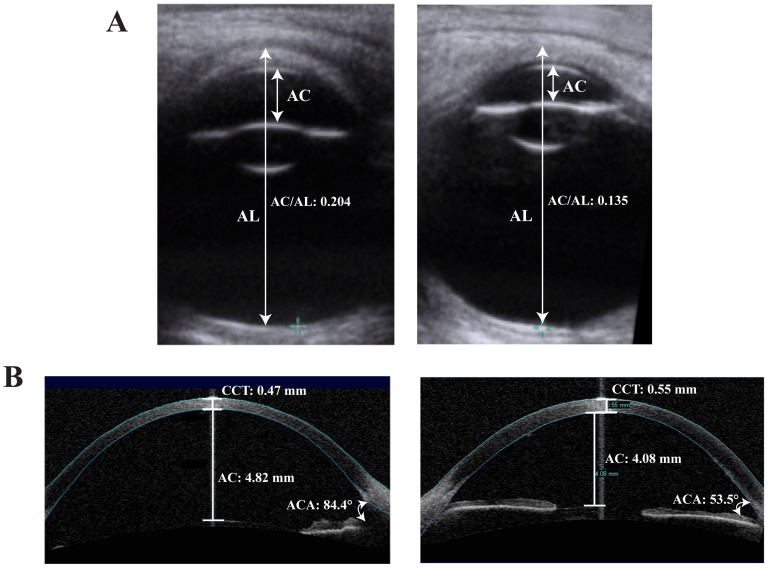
Comparison between biometry of X-linked megalocornea and congenital glaucoma. (A) AC, anterior chamber depth (back of cornea to front of lens); AL, axial length (anterior corneal surface to vitreo-retinal interface at the back of the macular). Transverse B-Mode ultrasound scans demonstrate higher ratio of the AC to AL in megalocornea, 0.204 (left) than congenital glaucoma, 0.135 (right). (B) Ocular coherence tomography (OCT) images comparing megalocornea (left) and arrested congenital glaucoma (right). Although there is enlargement of the anterior segment in both conditions, patients with megalocornea tend to have a greater anterior chamber (AC) depth, lower central corneal thickness (CCT), and deeper anterior chamber angles (ACA) than congenital glaucoma patients.
